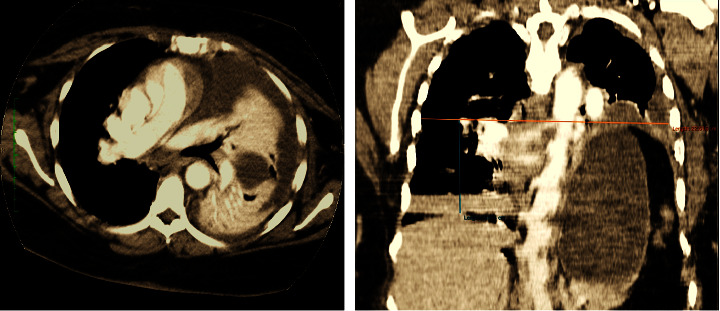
Axial and coronal reformatted CT scan images of the chest, mediastinal window. The images show dense consolidation with air bronchograms in the left lower lobe consistent with pneumonia. There is associated significant fluid in the left pleural space, in keeping with parapneumonic effusion. The coronal image shows ∼7.8 cm elevation of the left hemidiaphragm relative to the right hemidiaphragm, resulting in severe left lung volume loss and mediastinal shift to the right.
